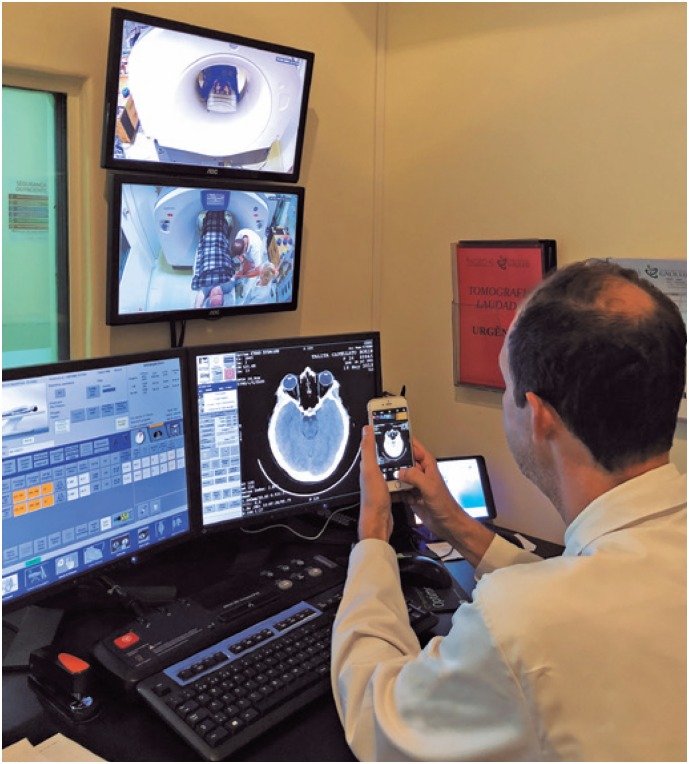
Obtaining computed tomography scan images of the skull
